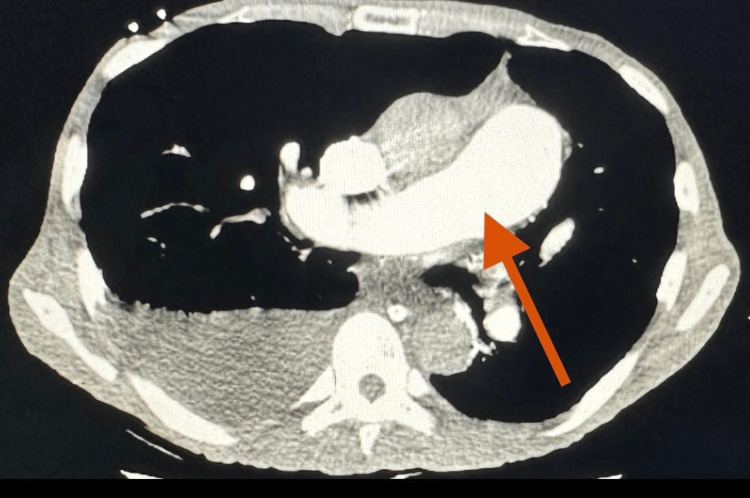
CTA chest showing dilation of pulmonary artery (red arrow) CTA: computed tomography angiography.
